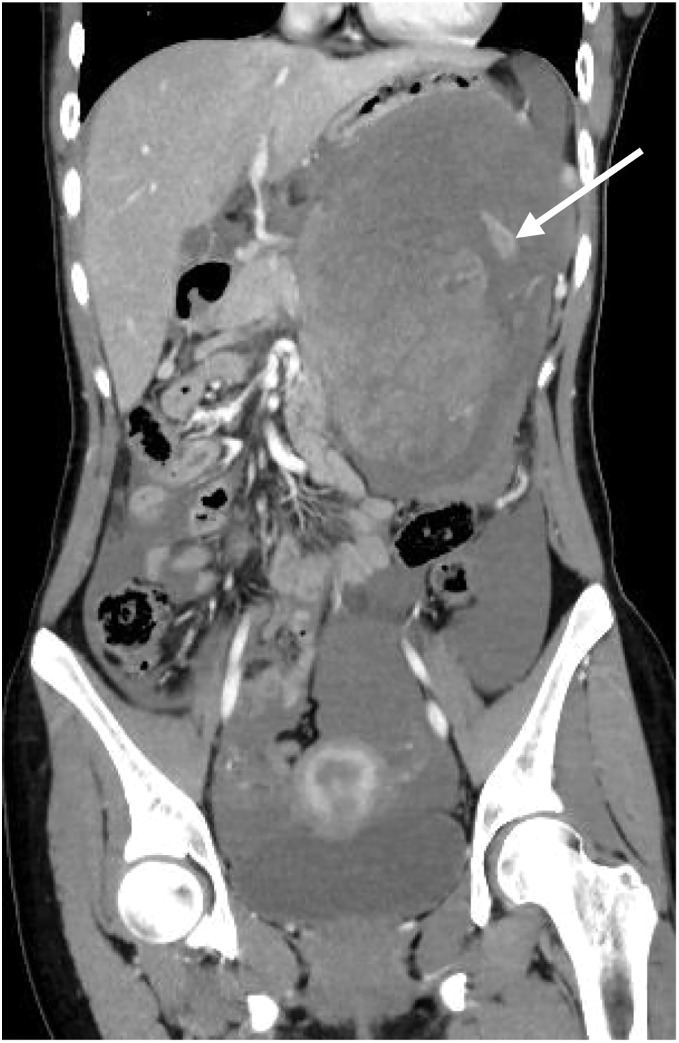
The tumor is heterogeneous and shows extravasation inside it (white arrow). CT shows massive ascites, suggesting intraperitoneal hemorrhage.
